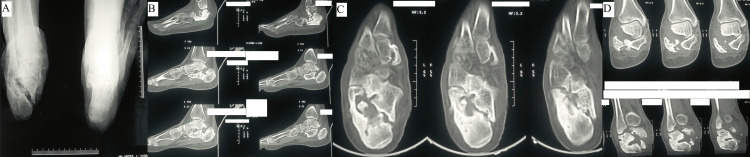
Radiographs and CT scan of the patient with associated nonunion of the calcaneus
